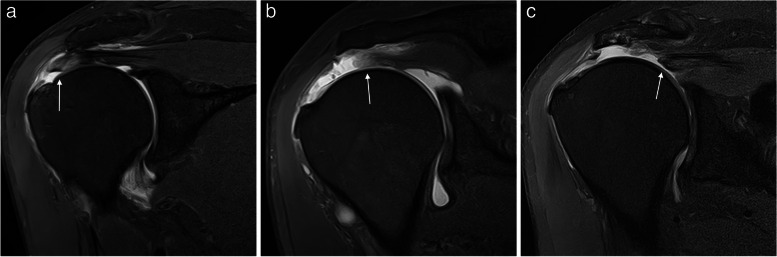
Supraspinatus full thickness tears different retraction stages. a, b Oblique coronal fat-suppressed PD-weighted MRI arthrography showing a complete full thickness tear with a small retraction (arrow in a) and a full thickness tear with fibers retracted over the humeral head vertex (arrow in b). c Oblique coronal fat-suppressed PD-weighted MRI shows tendon fibers retracted over the superior glenoid margin (arrow)
